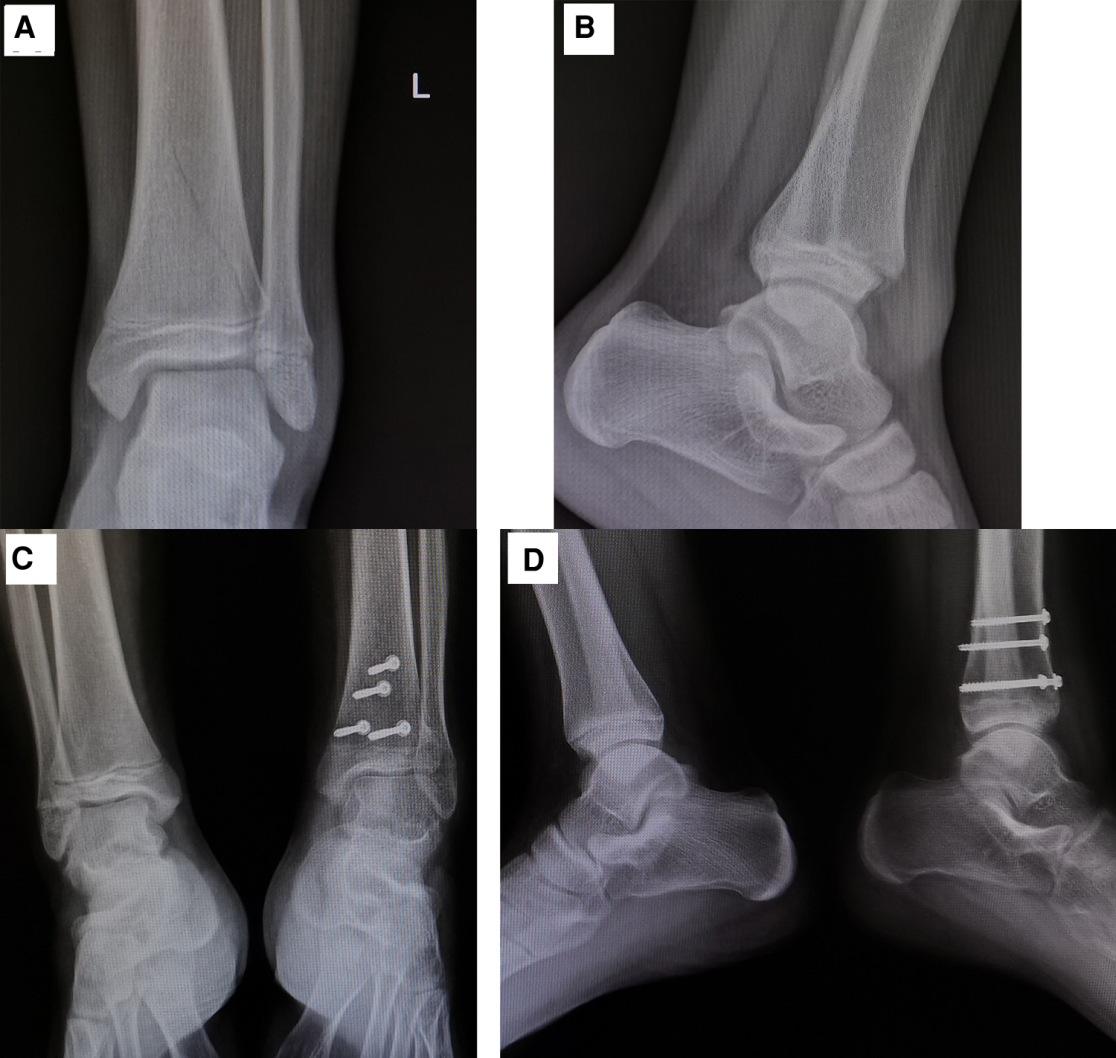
(A) Radiographs of a 13-year-old boy. The anteroposterior view of the left ankle joint shows the fracture line extending to the epiphysis. (B) Lateral view showing the fracture line extending from the posterior side of the tibial shaft to the anterior side of the epiphysis. The distal part of the epiphysis is displaced backward. This was classified as a Salter–Harris type II fracture. (C) At 6 months after the injury, the anteroposterior view of bilateral ankle joints shows no growth arrest lines. The epiphyseal plate of the affected side was blurred, while the epiphyseal plate of the contralateral side was still visible. This was judged as premature epiphyseal closure. (D) Lateral view of bilateral ankle joints showing also premature closure of the epiphyseal plate of the affected side.
